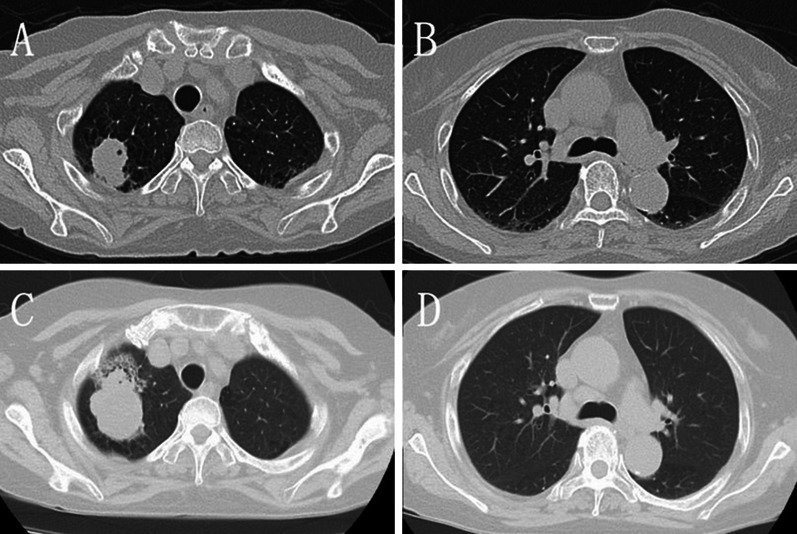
A Chest computed tomography (CT) scan shows a lobular mass of 33 mm located in the right upper lobe and B lymph node enlargement in station #4R. C Chest CT scan, taken 1 month after consultation, shows that the tumor diameter increased to 52 mm and D lymph node enlargement in station #4R
